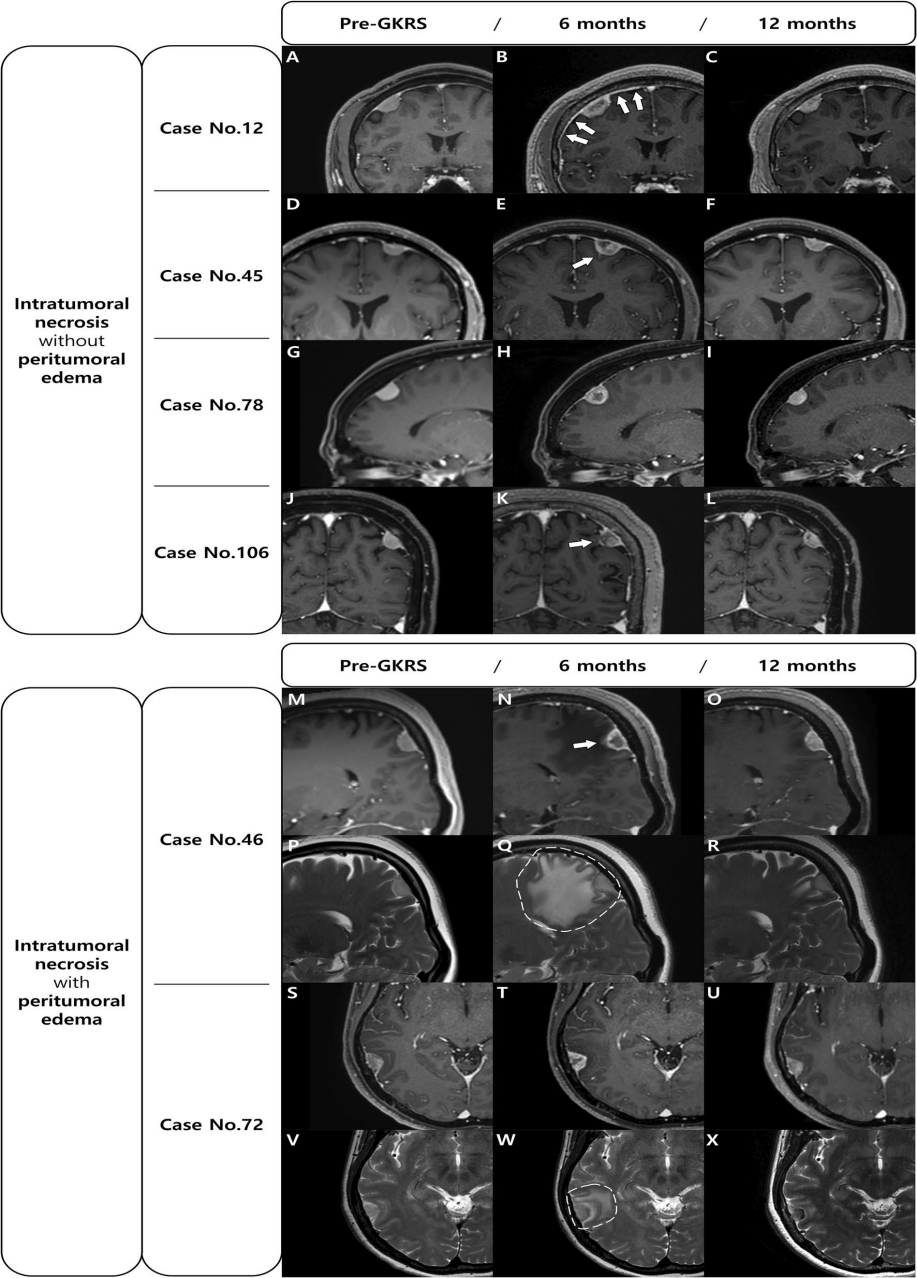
Changes occurring before and after GKRS are shown for 4 cases of ITN without PTE (A–L) and 2 cases of ITN with PTE (M–X). All meningiomas with ITN after GKRS show pseudoprogression with a temporary volume expansion on the follow-up MRI at 6 months (B,E,H,K,N,Q,T,W). ITN may accompany meningeal enhancement (arrows) on T1-weighted images and PTE on T2-weighted images (dotted circles). On the follow-up MRI at 12 months, the ITN has disappeared, the volume is reduced, and the enhancement and PTE are improved. *GKRS* gamma knife radiosurgery; *ITN* intratumoral necrosis, *PTE* peritumoral edema, *MRI* magnetic resonance imaging.
